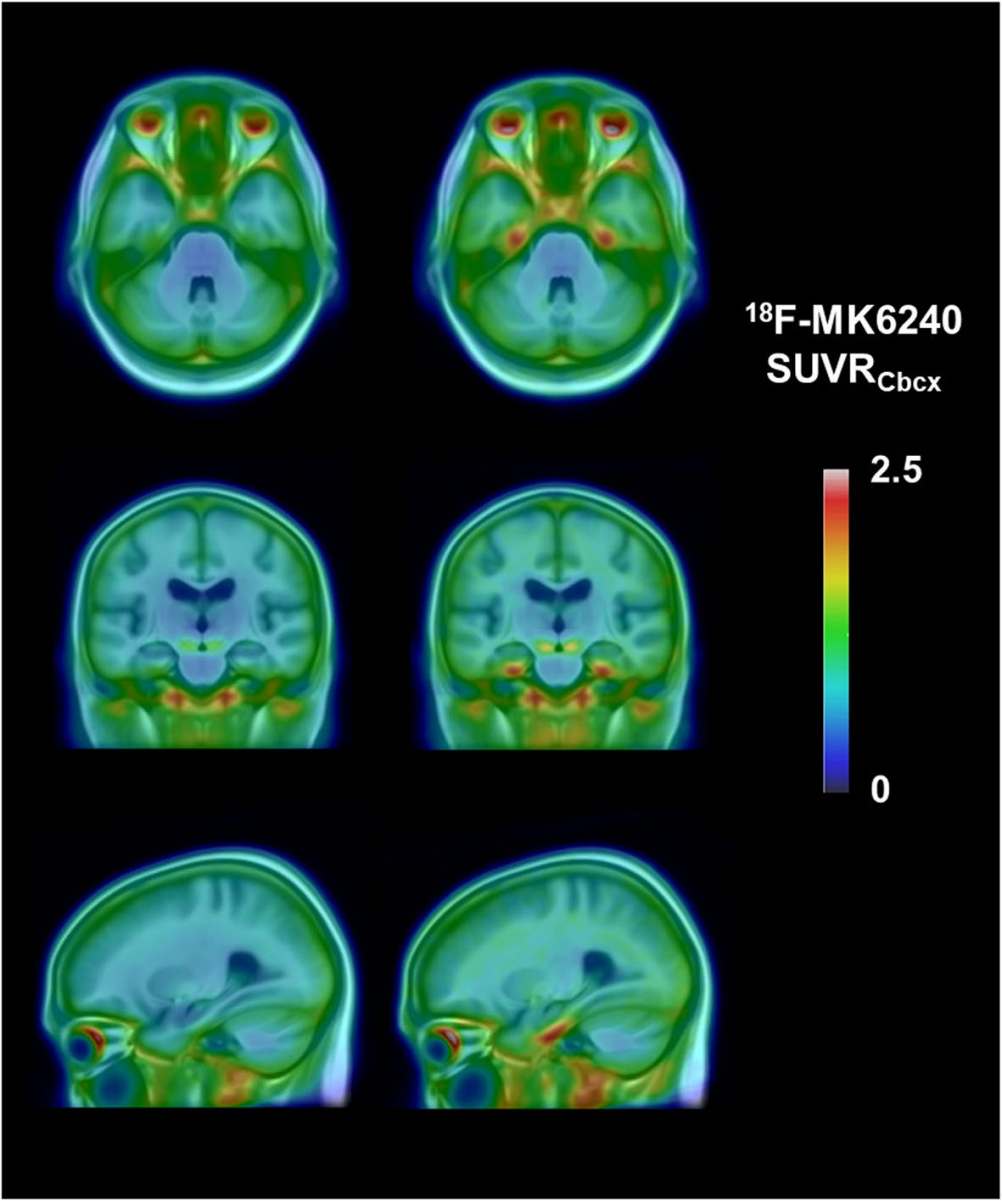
Mean tau ^18^F-MK6240 SUVR images for the cohort: lower 90% versus top 10%. Mean tau ^18^F-MK6240 SUVR images overlaid on a T1 MRI template for the cohort, lower 90% (left) and top 10% Me SUVR (right) showing tau tracer retention confined to Braak stages I–II
